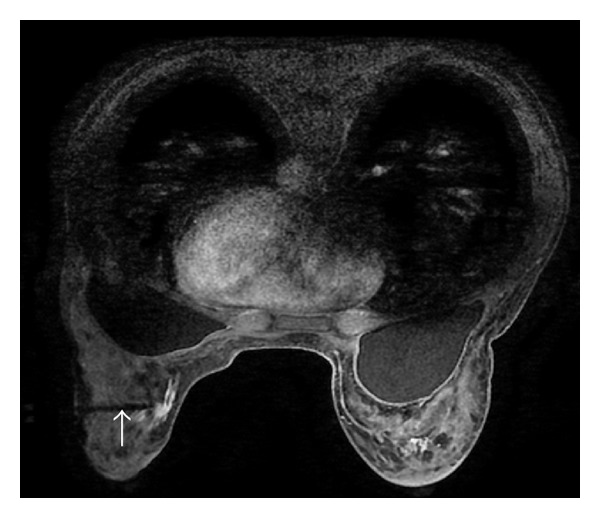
Axial postcontrast breast MRI in a patient who received silicone injection and mammoplastic bag placement shows a segmental enhanced lesion in left breast with a biopsy coaxial needle (white arrow) placed nearby. The biopsy diagnosis was adenosis.
